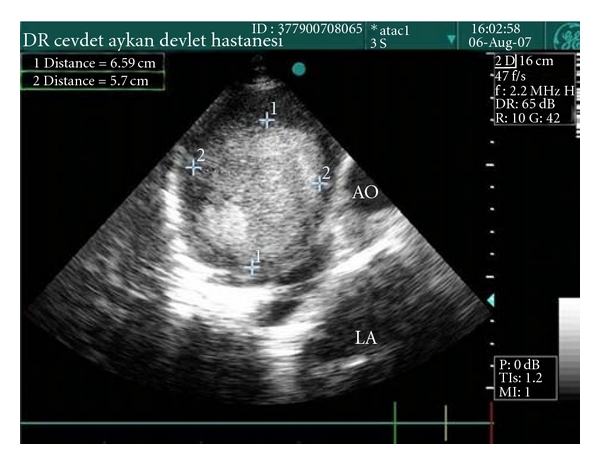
Paracardiac mass on transthoracic echocardiography.
